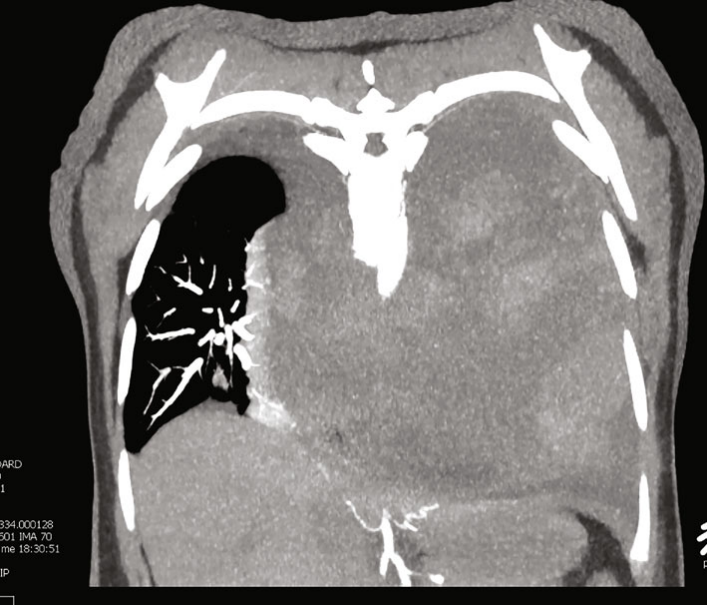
CT chest coronal MIP (maximum intensity projection) rightward mediastinal shift and partial right lung collapse secondary to left hemithoracic mass effect.
